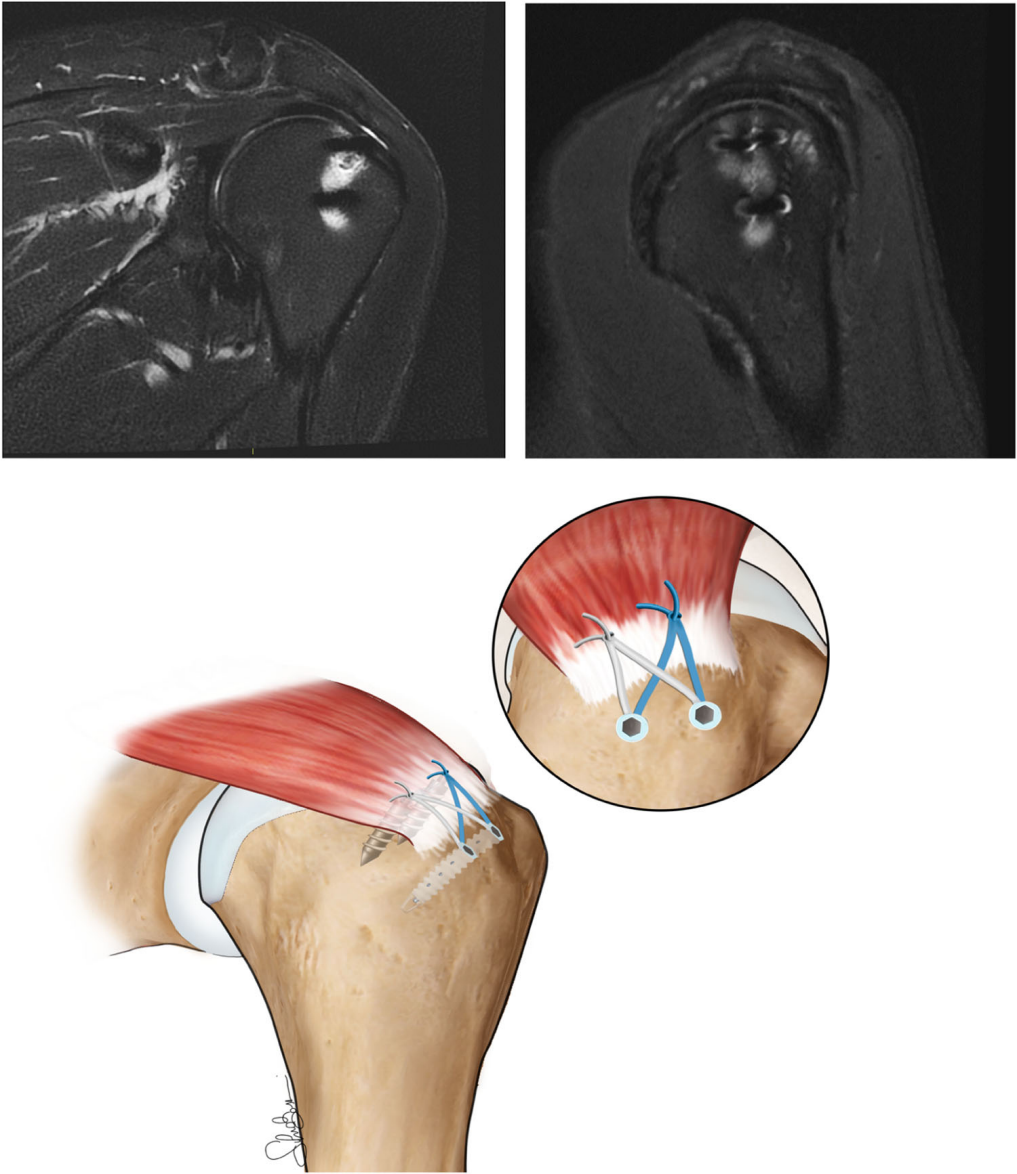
Double‐row suture‐bridge technique at 24 months minimum follow‐up. 3‐T MRI images show the supraspinatus tendon reattached to its footprint using two Corkscrew 5.5 mm metallic anchors preloaded with No. 2 Fiberwire sutures (Arthrex) and two footprint knotless anchors (SwiveLock SP 4.75 mm, Arthrex). The tendon appears continuous and well‐integrated. MRI, magnetic resonance imaging.
